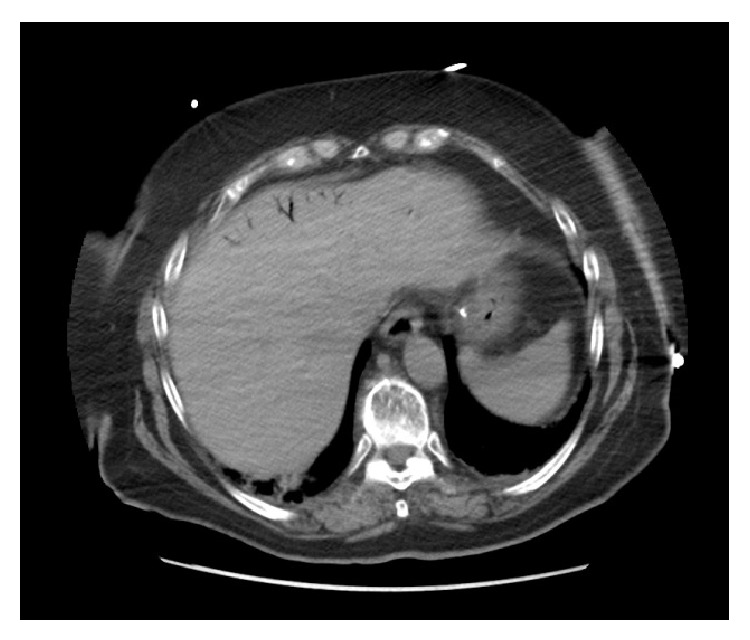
CT image demonstrating portal venous gas.
